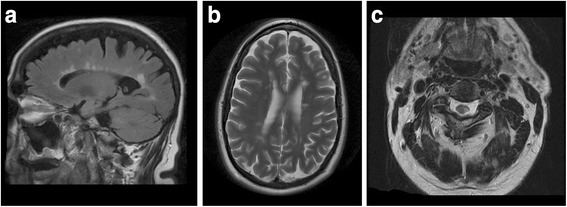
Magnetic resonance imaging of the brain. a A sagittal fluid attenuation inversion recovery sequence and b axial T2-weighted sequence demonstrate white matter lesions consistent with a diagnosis of multiple sclerosis. c A T2-weighted magnetic resonance image of his cervical spine demonstrates a lesion at the level of C3–4 affecting the left-sided dorsal horn and dorsolateral white matter tracts
